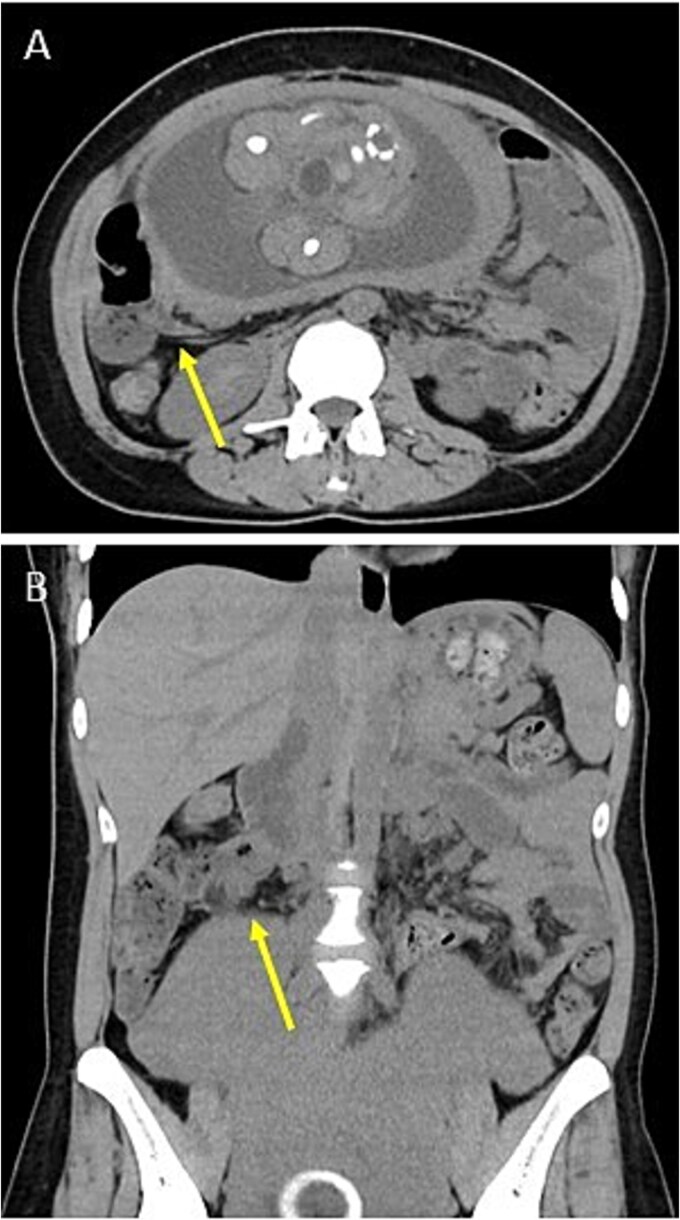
Preoperative images. Plain CT shows no appendix in the axial plane (A) or the coronal plane (B). Arrows indicate the terminal ileum.
